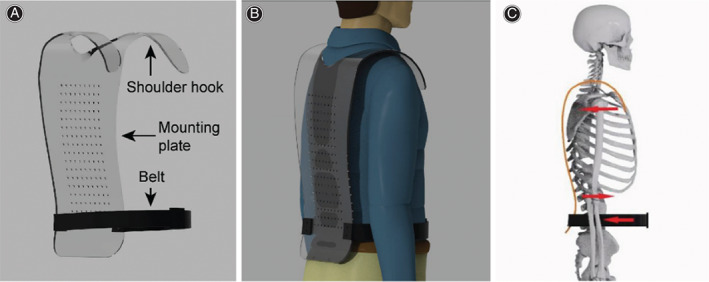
(A) The digital imaging and communications in medicine (DICOM) files were used for 3D reconstruction of the CT images, and then the 3D reconstruction model was transformed into STL files. (B) After the meshes and joints of the normal lumbar spine were set, solid modeling was conducted, and the ligaments were connected. (C) After the meshes and joints of the lumbar spine were set for L1 fractures, solid modeling was performed, and ligamentous connections (L1 anterior margin compressed by 20%) were made
